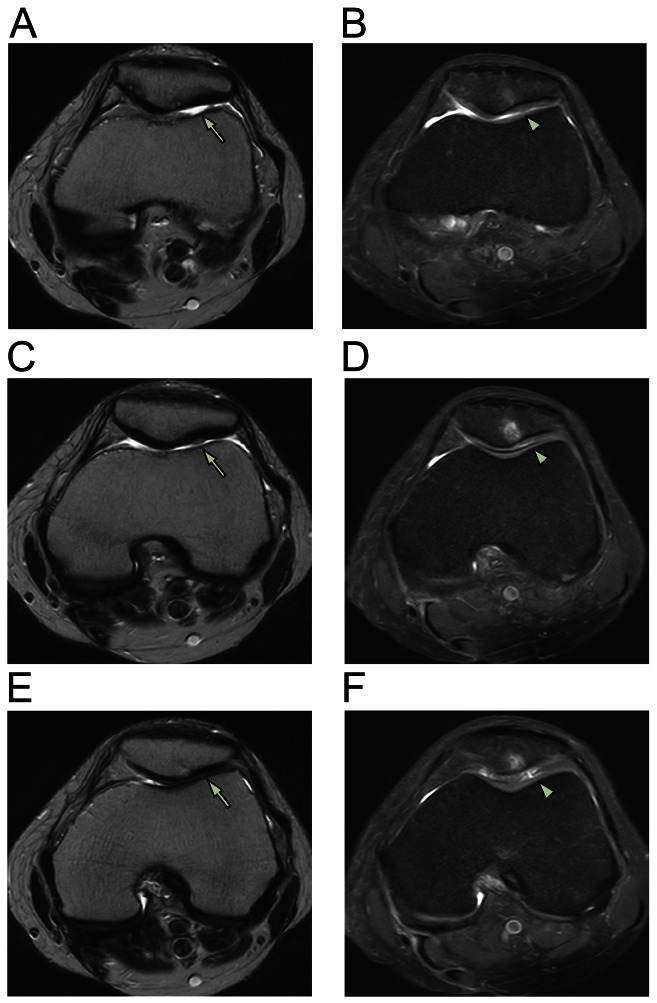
MRI axial sequential T2 views of patient 1. Pre-treatment MRI scans of patient 1 (A [sequential image: 3/16], C [4/16], and E [5/16]) show retro-patellar signal changes (arrow) consistent with chondromalacia patellae (upper bone). At three months, post-treatment MRI scans of patient 1 (B [6/20], D [7/20], and F [8/20]) show changes (arrowhead) consistent with probable cartilage restoration on the patellae-femoral joint.
